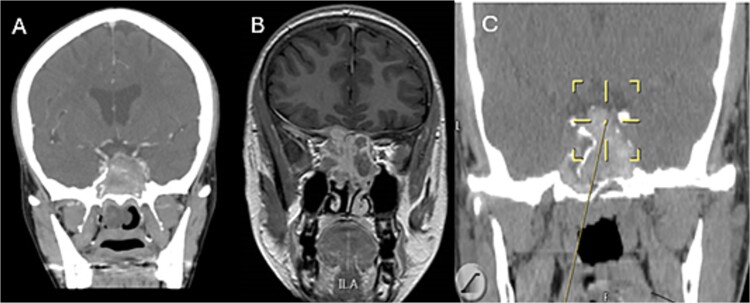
Coronal CT (A) and MRI (B) and CT image guided intra-operative (C) images of the paranasal sinuses showing aggressive sphenoid sinus disease extending to the skull base and cavernous sinus.
